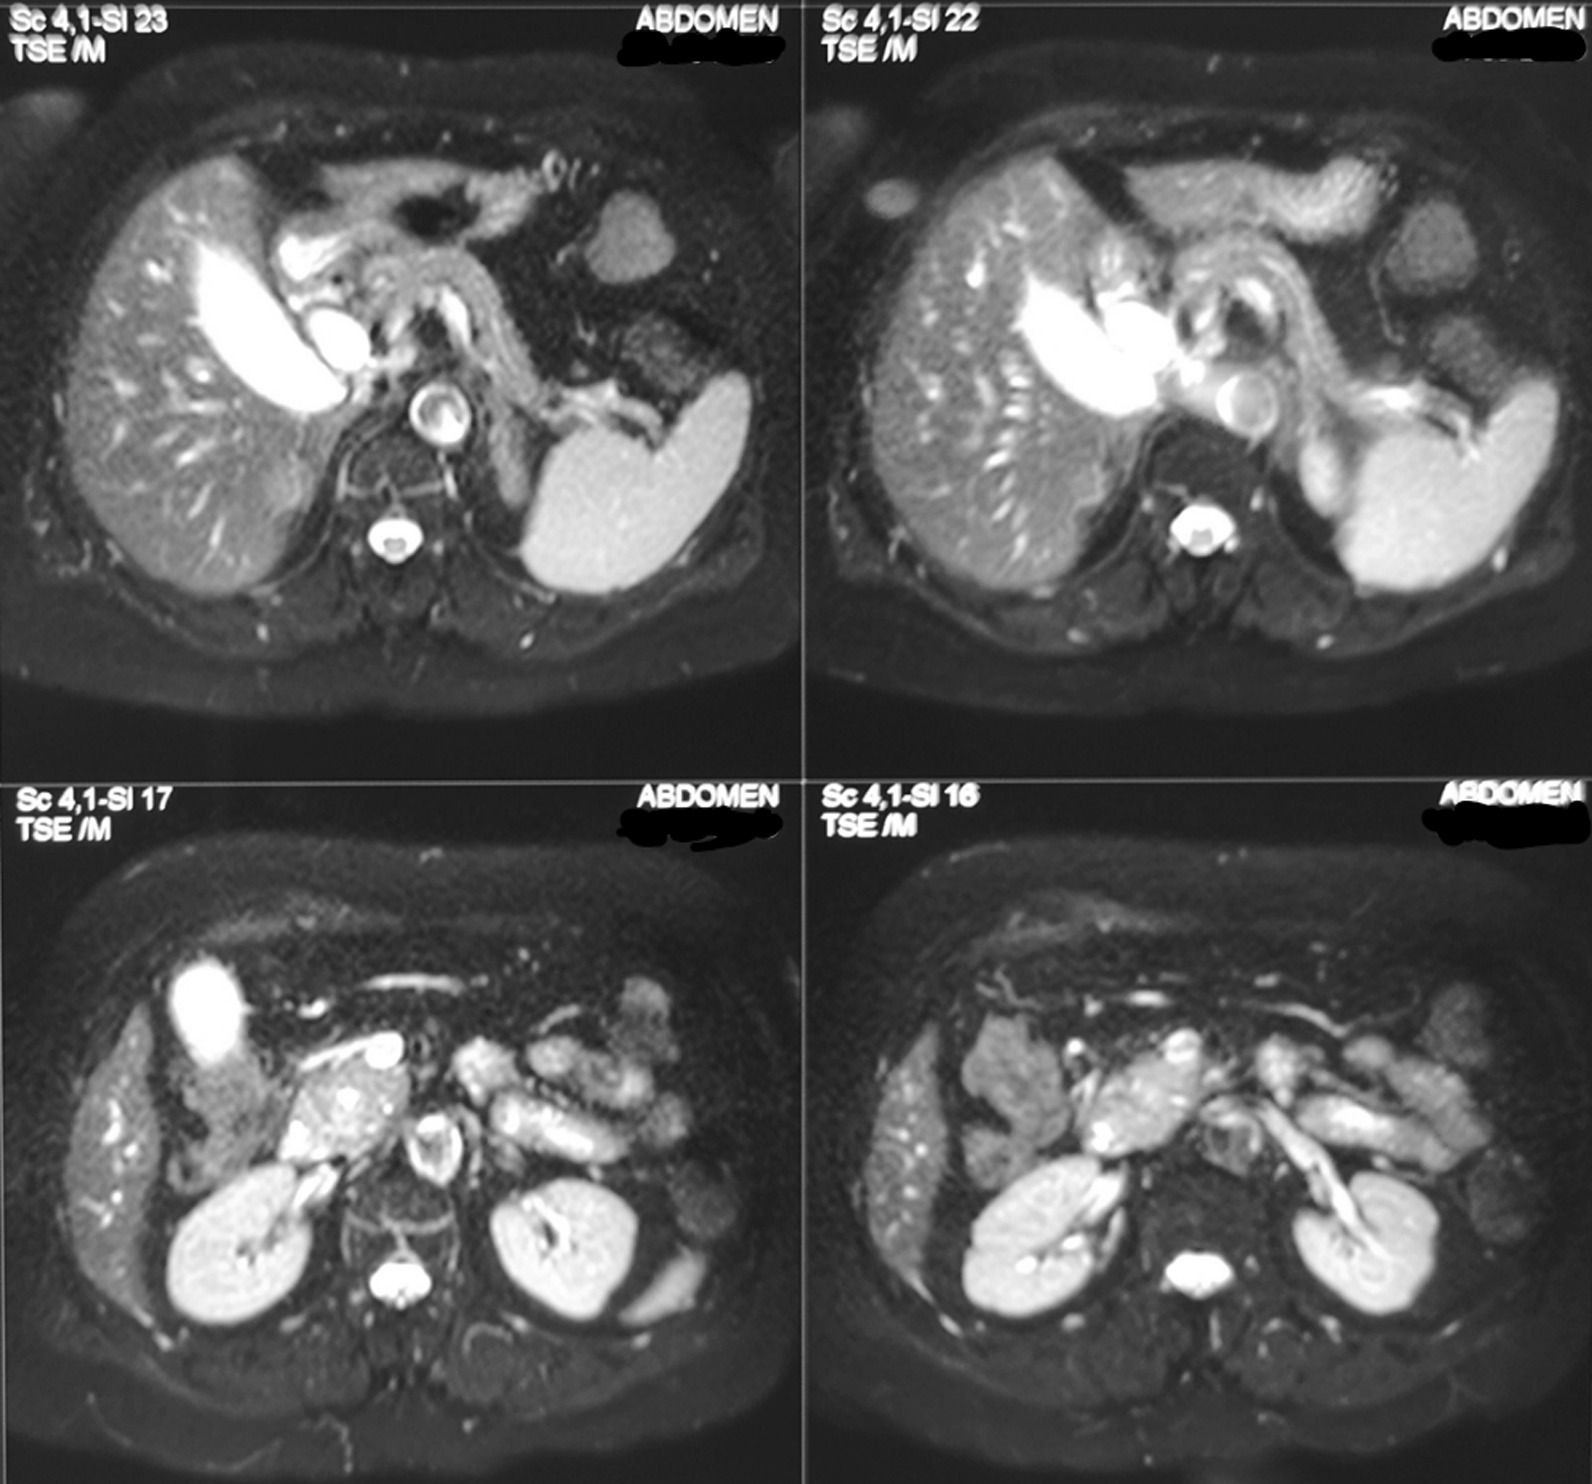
Magnetic resonance imaging image of the pancreatic tumor. Patient 1
